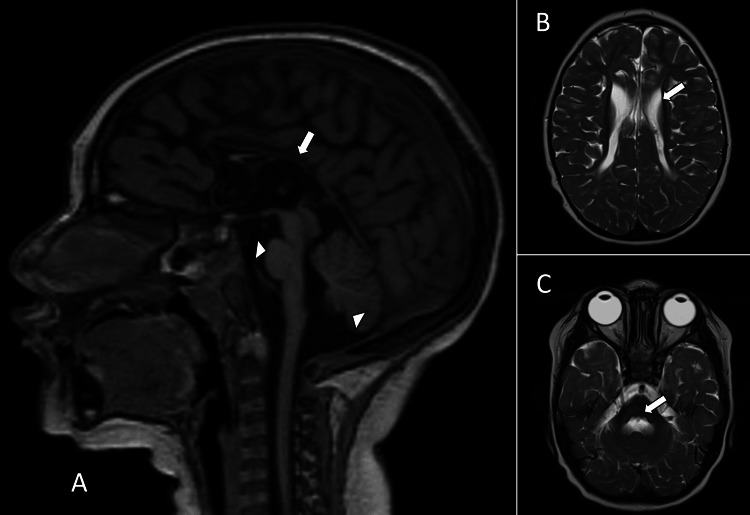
Brain magnetic resonance imaging without contrast administration (A) Sagittal T1-weighted volumetric image shows a decrease in the size of the cerebellum and less in the pons (white arrowheads) and hypoplasia of the corpus callosum (white arrow). (B) Axial T2-weighted image demonstrates significant loss of the periventricular white matter (arrow). (C) Axial T2-weighted image demonstrates pontine atrophy (white arrow).
